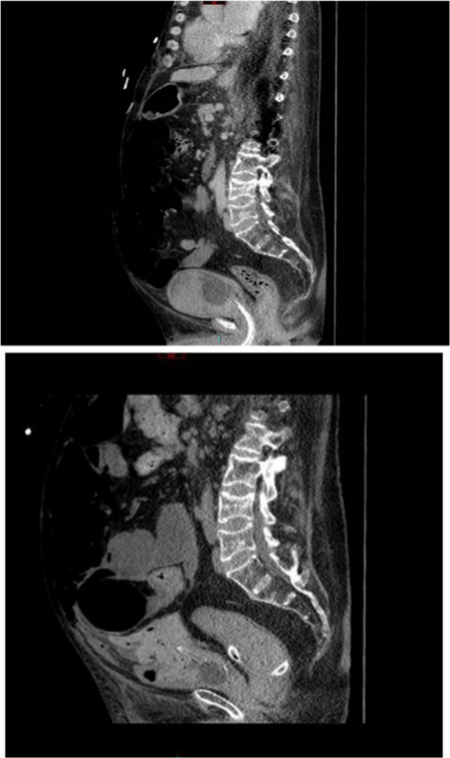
Top panel: Sagittal section of CT of abdomen performed five days after vesical lithotripsy showed urinary bladder distended with clots. But the outline of urinary bladder was intact. Bottom panel: Sagittal section of CT abdomen with oral positive contrast, performed 12 days after vesical lithotripsy (two days after cystoscopy and clot evacuation) when the patient developed abdominal distension: A large intra-peritoneal haematoma, at the superior aspect of dome of urinary bladder was seen which was in continuity with the lumen of the bladder. The tip of Foley catheter was abutting on the haematoma.
